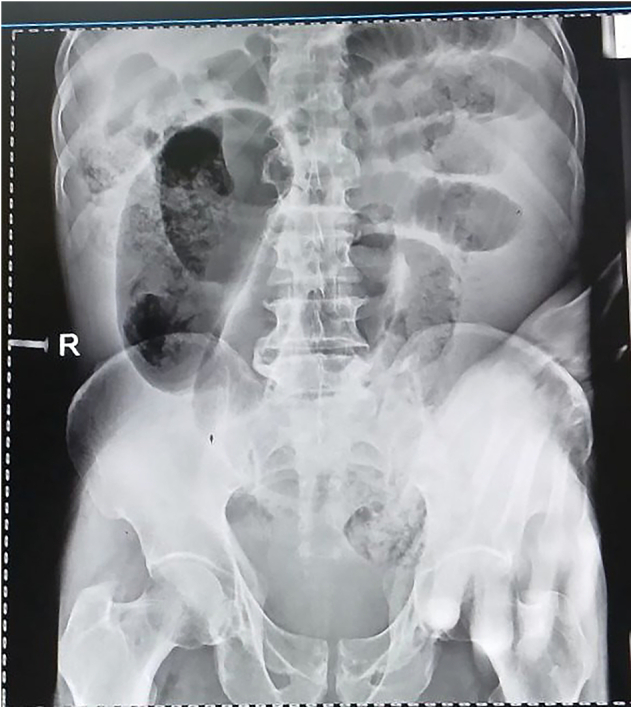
Abdominal X-ray demonstrated marked distention of a large bowel loop extends from the right lower quadrant to the left upper quadrant. Multiple dilated, centrally located small bowel loops with valvulae conniventes spanning their diameter are also noted, consistent with upstream small bowel dilation. No free intraperitoneal air or air-fluid levels are present.
